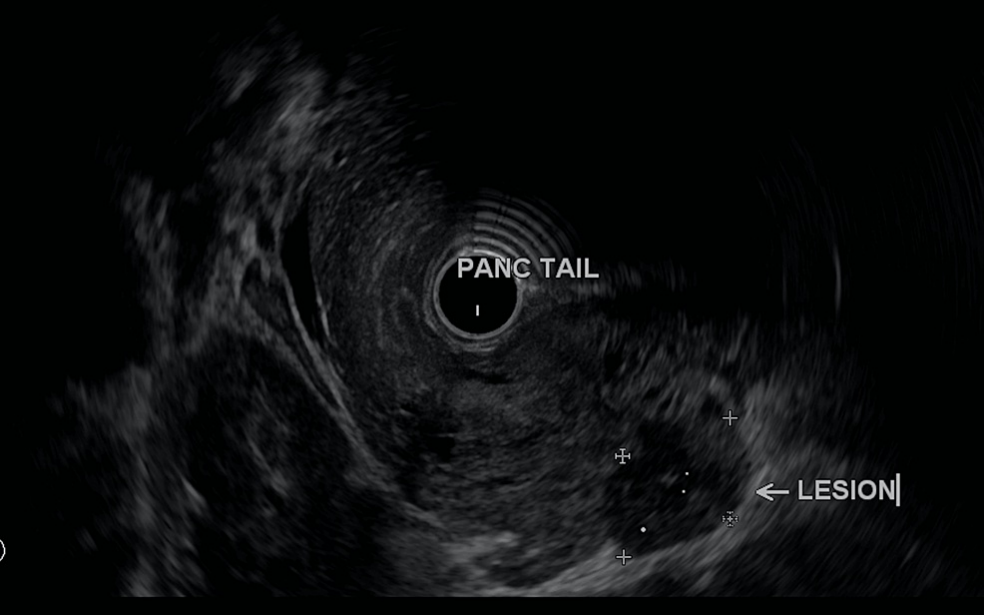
EUS exhibiting a hypoechoic 3.3 cm mass at the pancreatic tail (white arrow) EUS: endoscopic ultrasound
